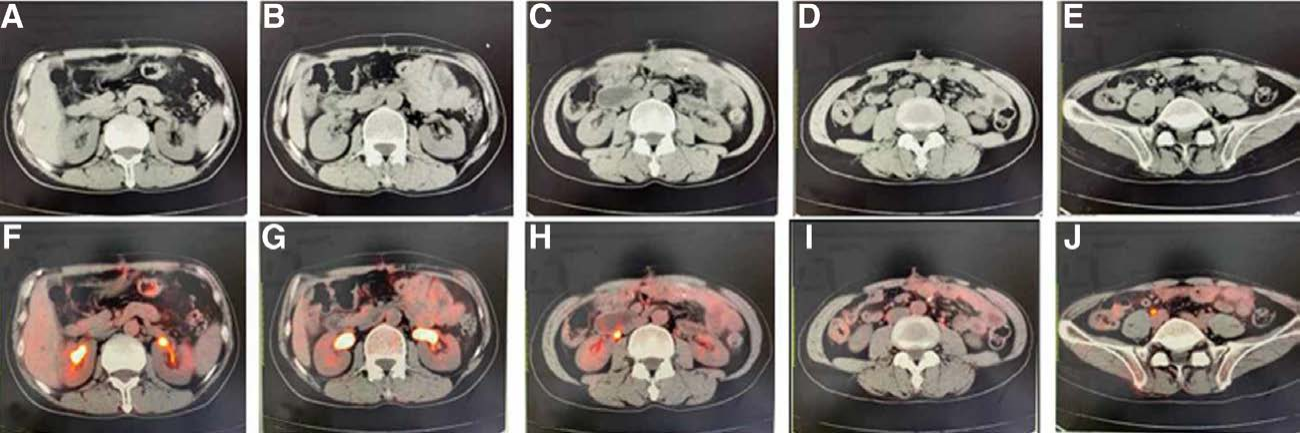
(A–J): PET-CT: no abnormal radioactive distribution was found at the corresponding place. Cord shadow can be seen under the skin of the abdominal wall operation area, with slightly high radiation distribution, and the highest SUV is 1.5. PET-CT = positron emission computed tomography.
